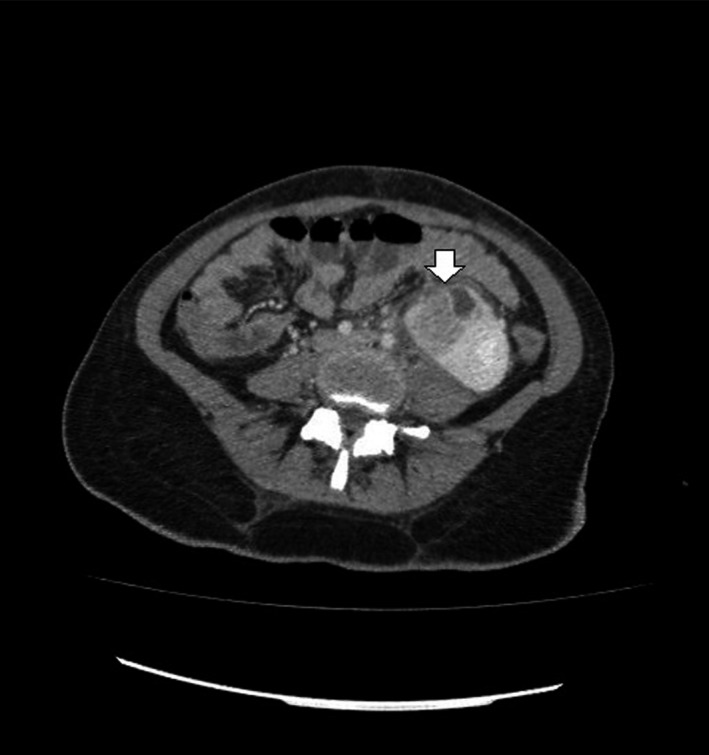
The abdominal CT scan which was performed after the initial assessment of the patient with the US confirmed the diagnosis of the left pelvic renal ectopia and the abscess formation in its upper pole (The arrow indicates the site of the abscess)
